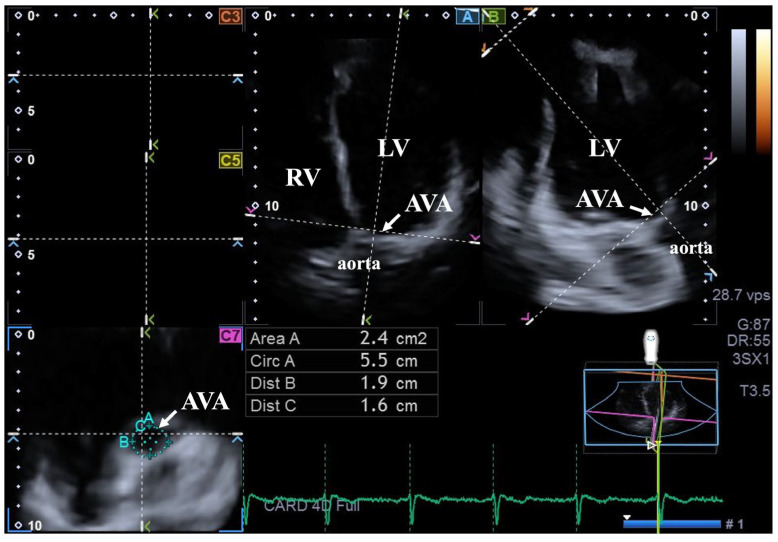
Assessment of aortic valve annular dimensions using three-dimensional speckle-tracking echocardiography. (A) Apical four-chamber and (B) apical two-chamber long-axis views, alongside a (C) cross-sectional view of the aortic valve annulus optimized from images (A) and (B). The aortic valve annular plane is indicated by white arrows. Abbreviations: LV: left ventricle; AVA: aortic valve annulus; Area: AVA area; Circ: AVA perimeter; Dist B: maximum AVA diameter; Dist C: minimum AVA diameter.
